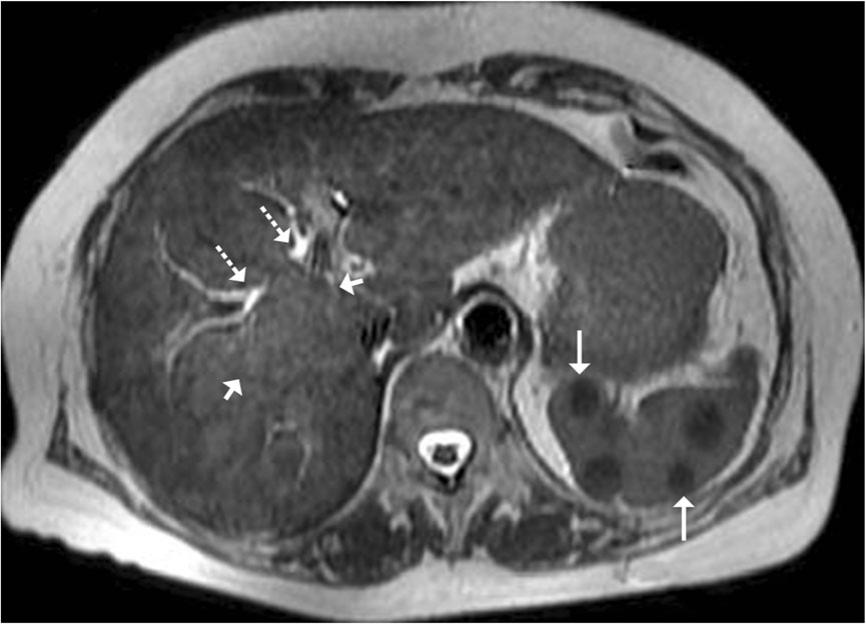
Axial T2-weighted MRI displaying an ill-defined heterogeneous area (*short arrows*) in the right lobe of liver corresponding to granulomatous infiltration, which extends into the periportal location, resulting in central biliary radical dilatation (*dotted arrows*) with concurrent multiple hypointense splenic nodules (*arrows*) in a patient with hepatobiliary tuberculosis
